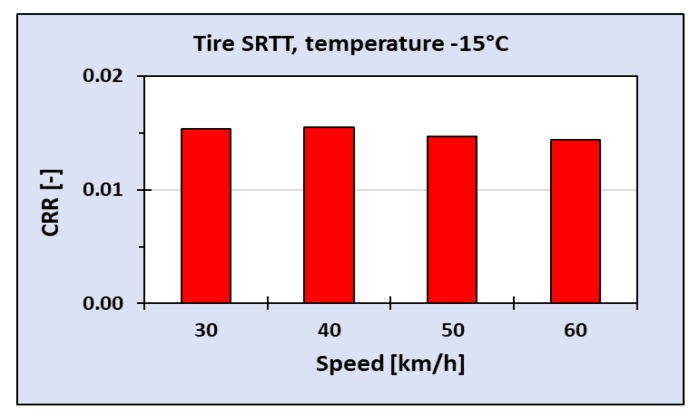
Coefficient of Rolling Resistance (CRR) for SEPOR-D-PSMA5 W4 as measured on the roadwheel facility at −15 °C.
